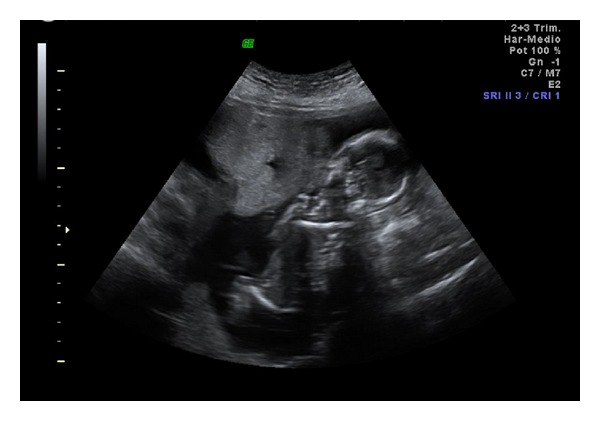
Abdominal ultrasound image showing the myoma in the front face of the uterus that clearly evidences the fetal compression. Both fetal legs and arms are in a hyperflexion position.
